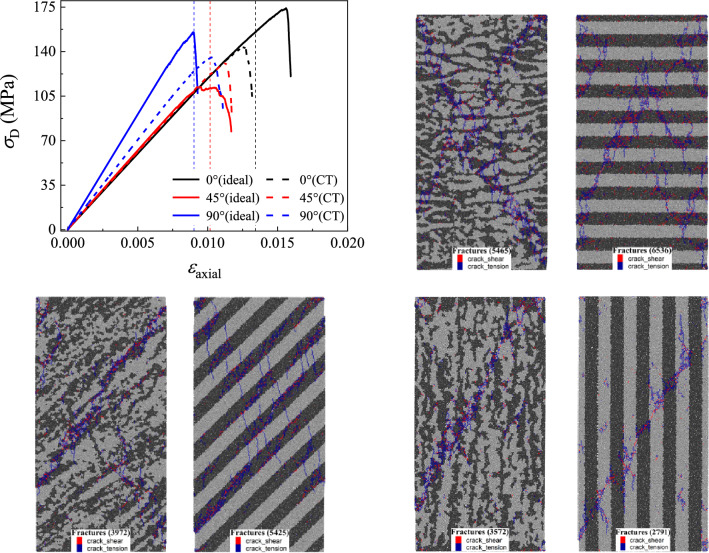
Comparison between the ideal and the CT models.
